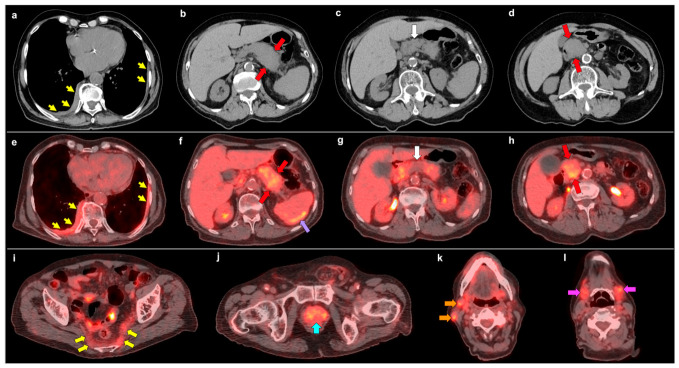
IgG4-RD with multifocal AIP and multiorgan involvement in an anicteric male patient, incidentally detected on non-contrast chest CT performed for persistent cough and recurrent bronchitis. (a–d) Axial chest CT images show band-like paravertebral soft tissue thickening along the right posterior pleura, and mild thickening of the left pleura (a, yellow arrowheads). Inferior sections including the upper abdomen demonstrate enlargement of the pancreatic head, body and tail with loss of lobulations and a pseudomass appearance (b,d, red arrows), while the pancreatic neck remains preserved (c, white arrow). These findings raised suspicion of IgG4-RD with multifocal AIP and pleural involvement, prompting further evaluation with ^18^F-FDG PET/CT and MRI. (e–l) ^18^F-FDG PET/CT demonstrates intense uptake in the pleural thickening (e, yellow arrowheads) and in the pancreatic head, body, and tail (f,h, red arrows), with sparing of the pancreatic neck (g, white arrow). Additional hypermetabolic foci are identified in the perisplenic tissue (f, purple arrow), presacral retroperitoneal fat (i, yellow arrows), prostate (j, cyan arrow), cervical lymph nodes (k, orange arrows) and submandibular glands (l, pink arrows), consistent with multiorgan involvement secondary to IgG4-RD.
